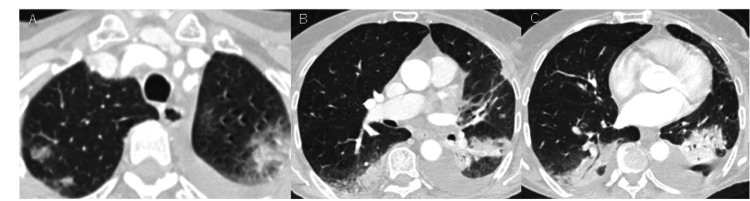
Chest CT on day 8 after admission. Extensive pulmonary condensations.
